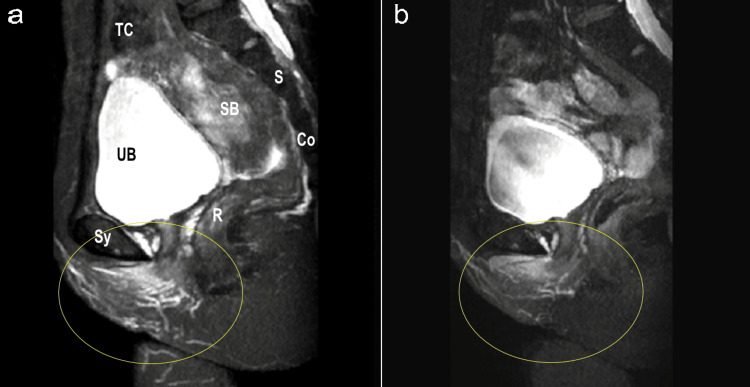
Changes in perineal congestion on heavy T2-weighted imaging a: before treatment; b: after treatment Sy: symphysis pubis; UB: bladder; SB: small intestine; R: rectum; S: sacrum; Co: coccyx; TC: transverse colon Yellow open circle: the venous congestion of blood
